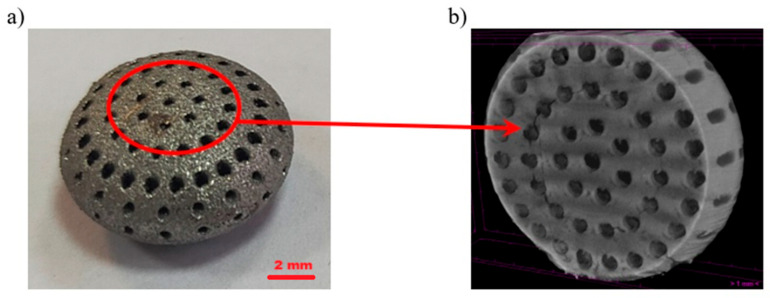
Capsule damage line under compression: (a) visible fracture line, (b) CT scan image indicating the damage line.
